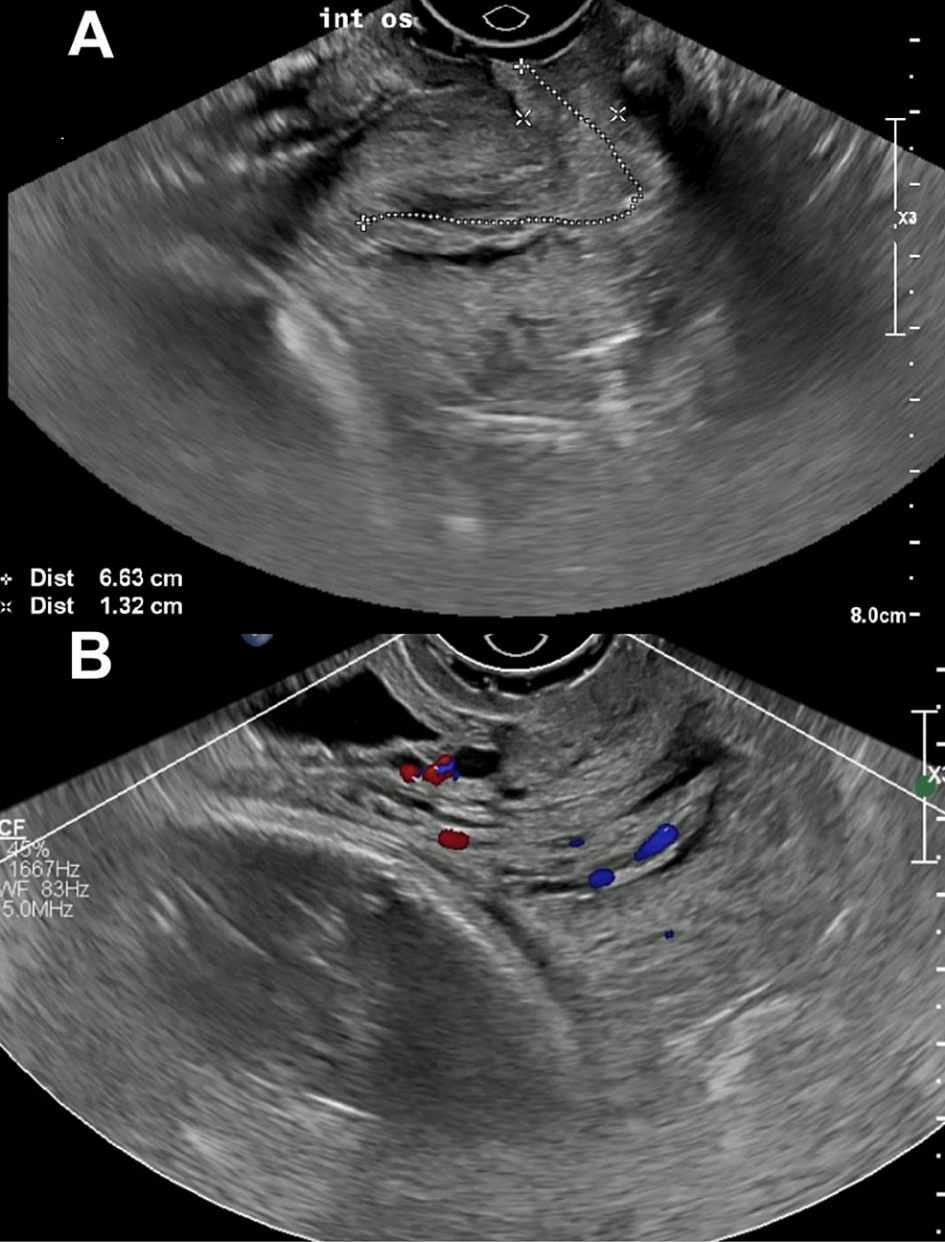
Transvaginal ultrasound. Transvaginal ultrasound showing grayscale imaging (A) and color Doppler imaging (B) of an endocervical polyp. CF: color flow, WF: wall filter, int os: internal opening of the cervix.
